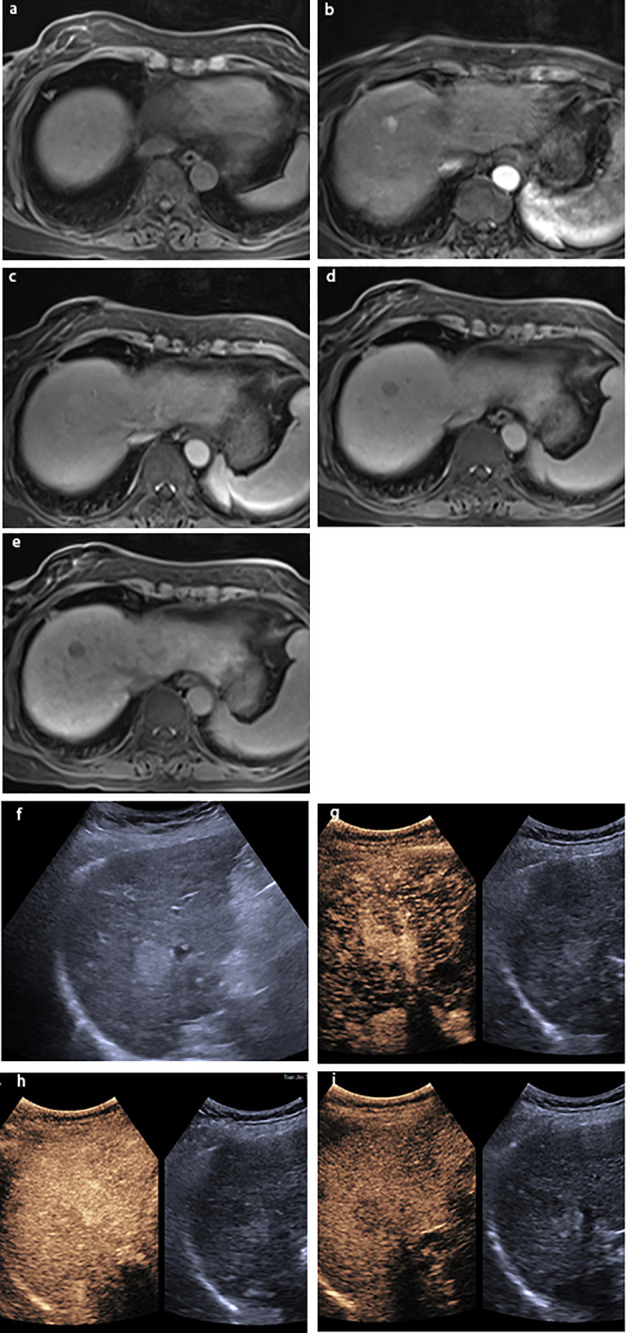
A lesion in S6 with a size of 2.0×1.6 cm in a 51-year-old man with chronic HBV infection and cirrhosis. The lesion was classified as LR-4 by EOB-MRI: (A) the lesion was hyperintensity on T1WI; showing (B) arterial phase slightly hyperenhancement, (C) hyperintensity on the portal venous phase, (D) isointensity on the transitional phase, and (E) hypointensity on the hepatobiliary phase; (F) gray-scale ultrasound found a lesion on S6. The lesion was classified as LRM by CEUS: showing (G) arterial phase hyperenhancement and (H) washout on 58 s. (I) The lesion was hypovascular on the delay phase. The pathological result by ultrasound-guided puncture was highly differentiated HCC.
